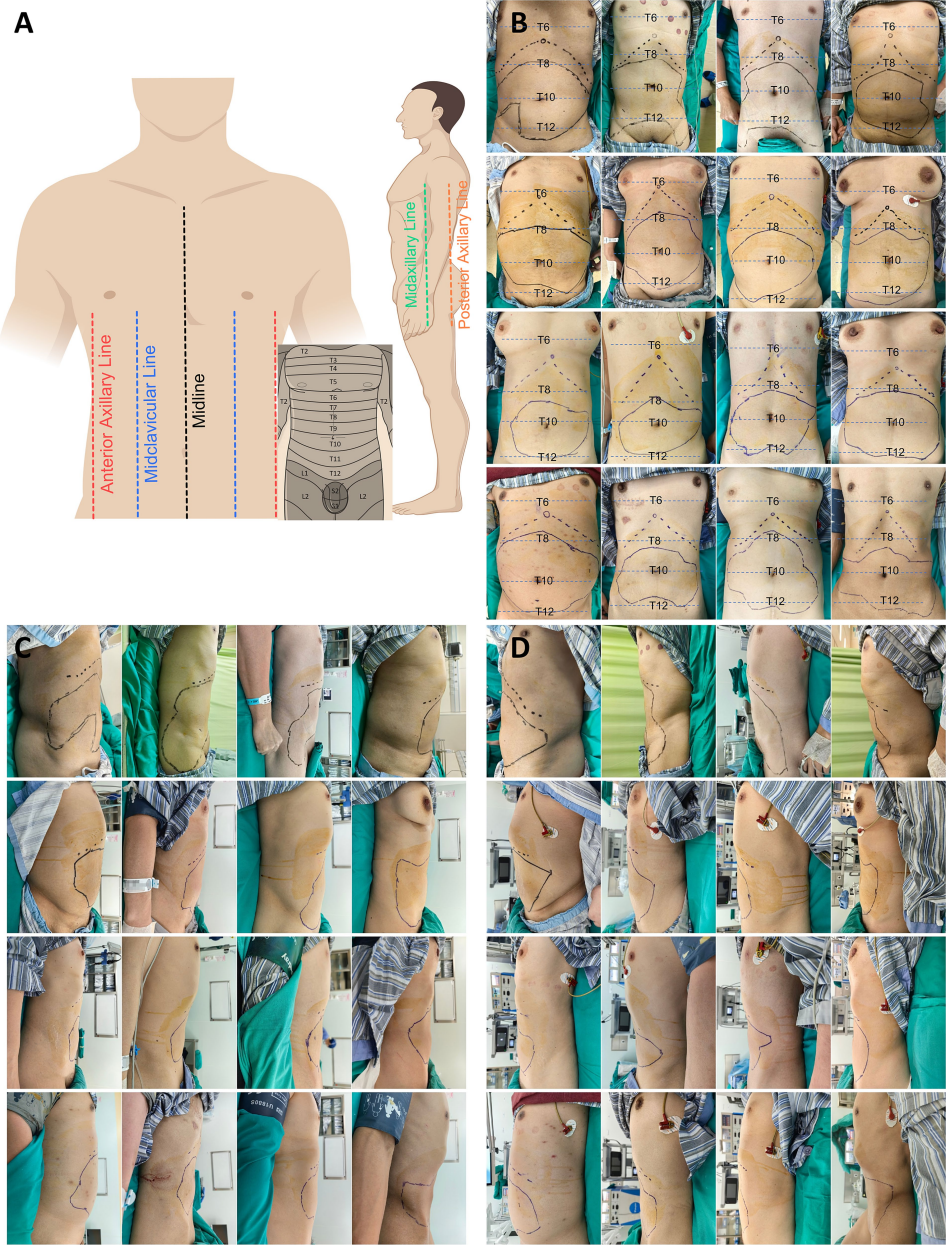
Visualisation of the cutaneous sensory block area (CSBA) after bilateral novel ultrasound-guided transversus abdominis plane block approach in 16 subjects. (A) Outline the skin areas of the anterior abdominal wall and anterolateral abdominal wall with vertical lines of different colors. (B) Frontal view. The solid line portion represents the CSBA, the dashed line represents the costal margin, and the circle represents the sternal angle. (C) Right lateral view; (D) left lateral view.
